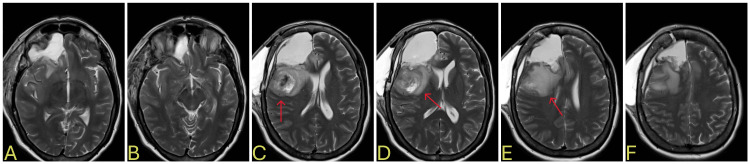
Brain MRI prior to the second admission, 19 days after the first resection A-B: postoperative changes and swelling 19 days after the first resection, C-F: a new mass formation that was not seen on previous MRI with midline shift (red arrow), significant enhancement at the lateral aspect of the resection cavity MRI: magnetic resonance imaging
